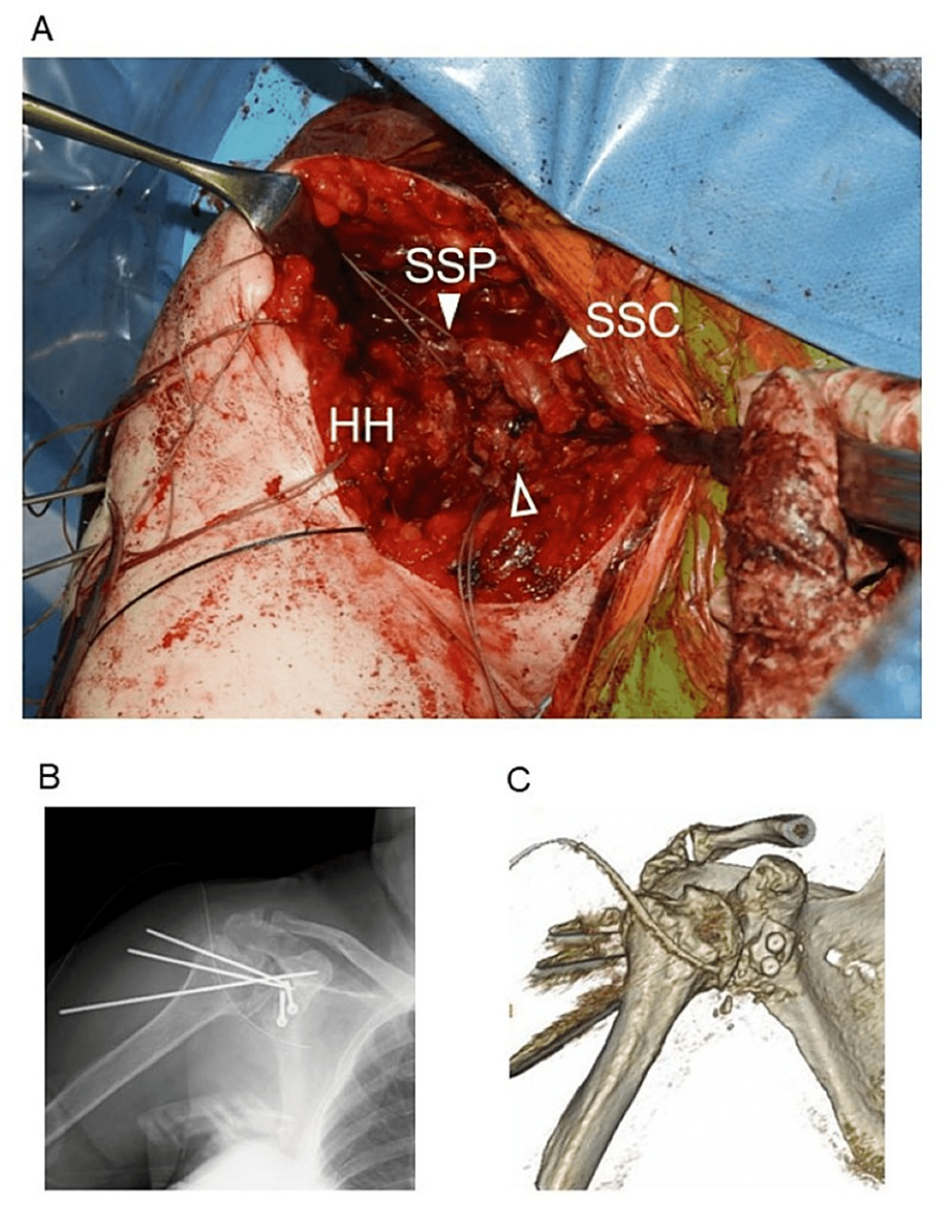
The perioperative images. A) The intraoperative photograph immediately following reduction of the humeral head and transfer of the coracoid to the glenoid. White arrowheads indicate full-thickness tear of the subscapularis and supraspinatus. The white outlined arrowhead indicates the transferred coracoid. SSP: supraspinatus, SSC: subscapularis, HH: humeral head. B, C) Postoperative X-ray and CT scan.
